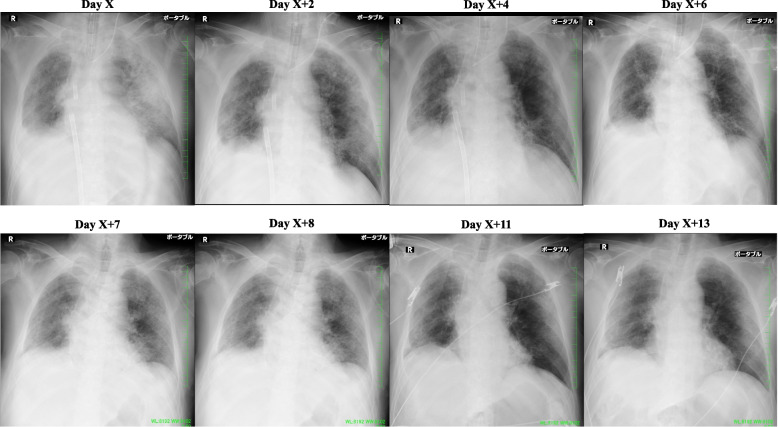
X-ray images showing the disease course of interstitial pneumonia in the patient after postoperative readmission to hospital. At the time of introduction of ECMO, bilateral frosted shadows and mild congestive images were detected, partly due to the effect of infusion. With the course of treatment, the frosted shadows tended to disappear and the congestive images improved. After extubation, a slight deterioration was observed, but the patient showed a tendency toward improvement (day X + 7)
